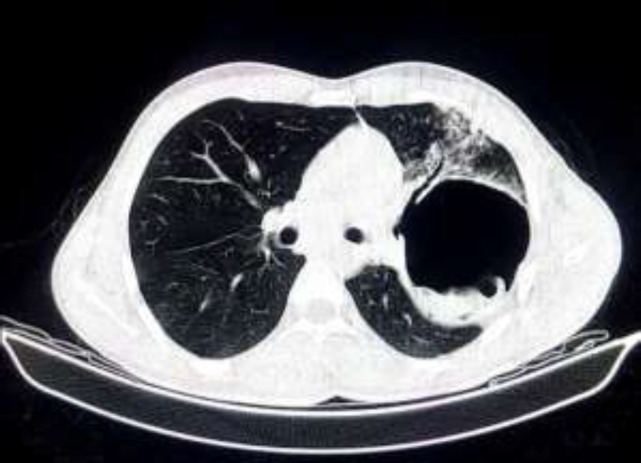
Computed tomography of the chest in 36-year-old male patient presented with history of severe coughing, dyspnea, and productive salty sputum. Note the ruptured cysts into the left lobar bronchus
